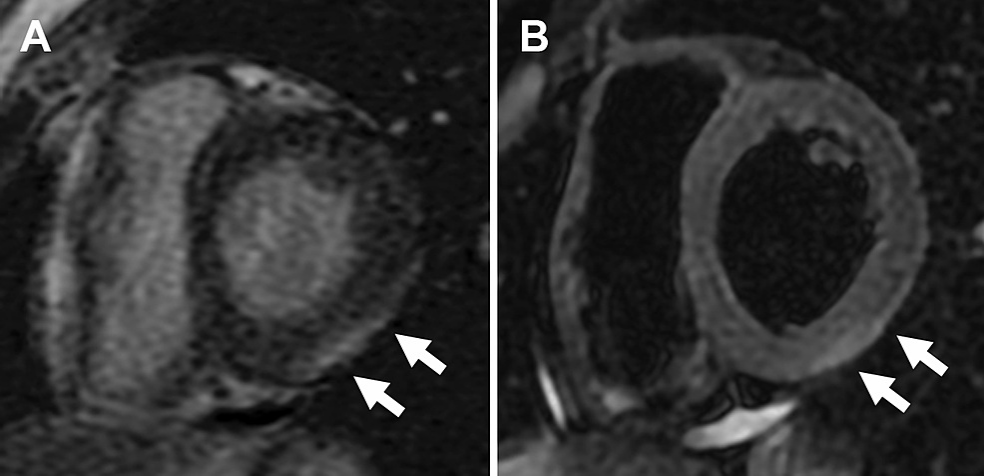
Cardiac magnetic resonance imaging on admission. (A) Sub-epicardial late gadolinium enhancement presents in the mid-inferolateral segments on the left ventricle (arrows). (B) T2-weighted images showing elevated T2 values in the mid-inferolateral segments on the left ventricle (arrows).
